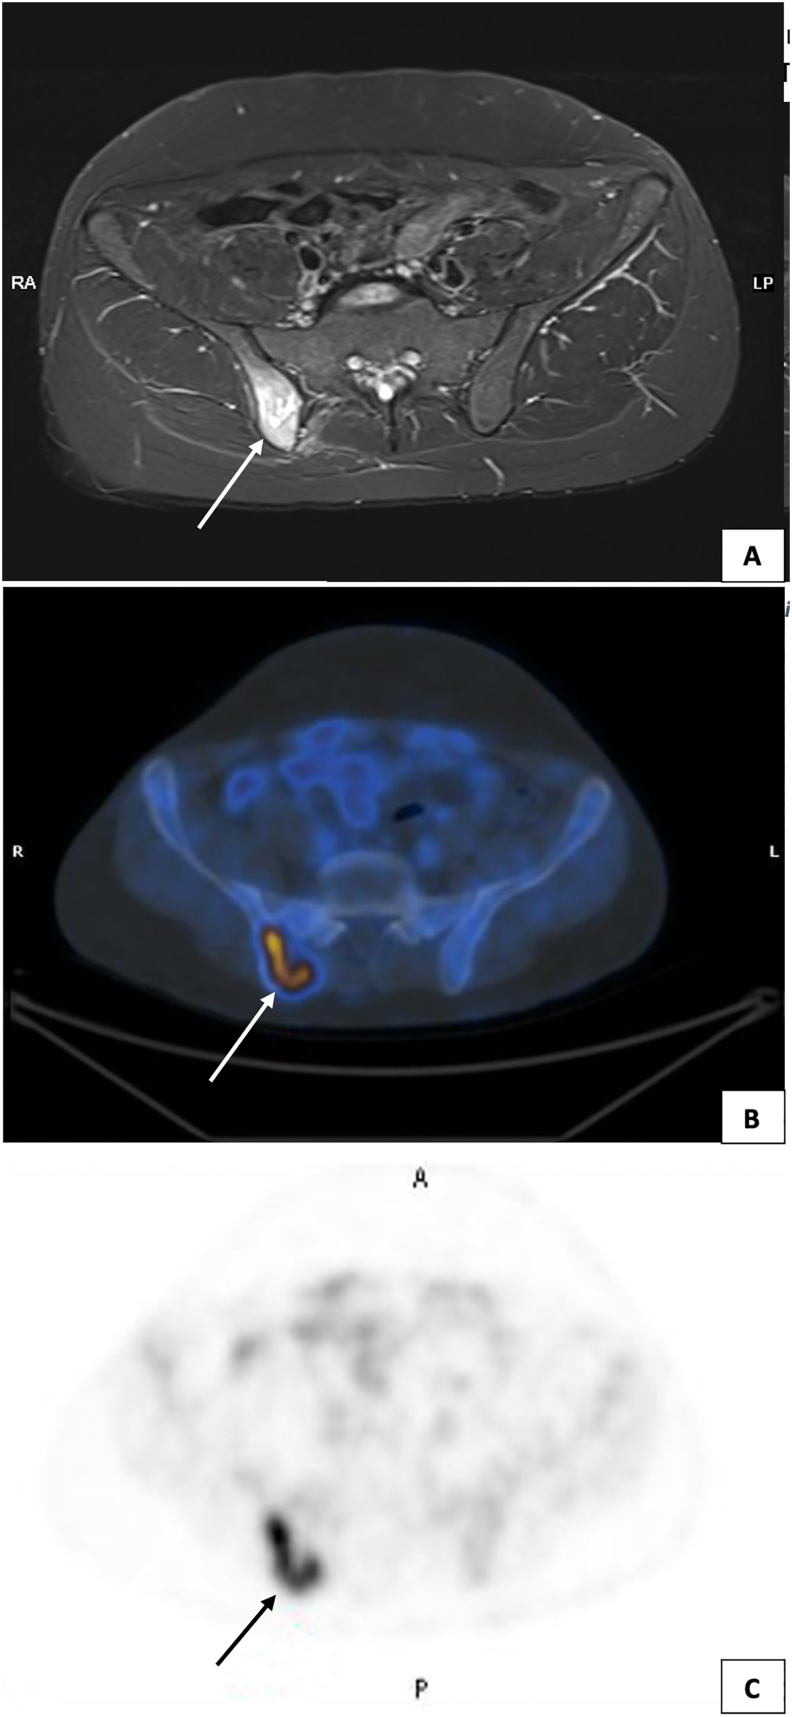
(A) MRI Pelvis with T2 STIR sequence showing increased signal intensity in the right posterior inferior iliac spine. (B–C) PET CT scan showing uptake of 18-FDG.
